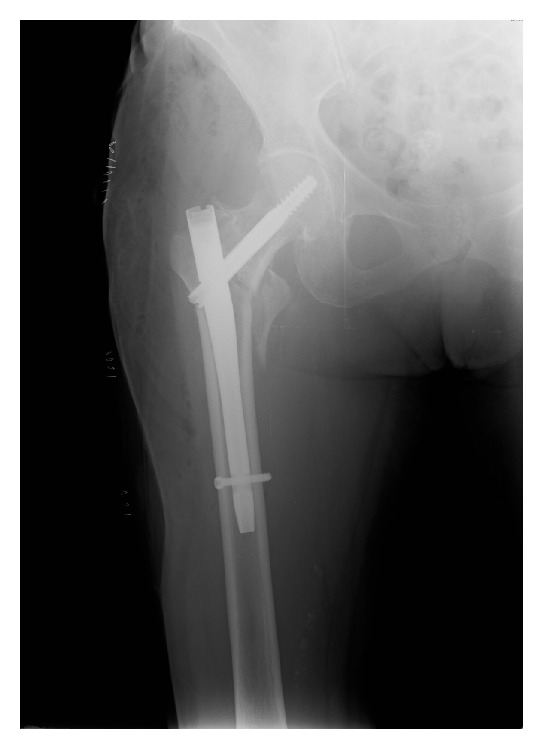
Immediate postoperative AP view radiograph of the right hip demonstrating good position of the anterograde intramedullary nail.
